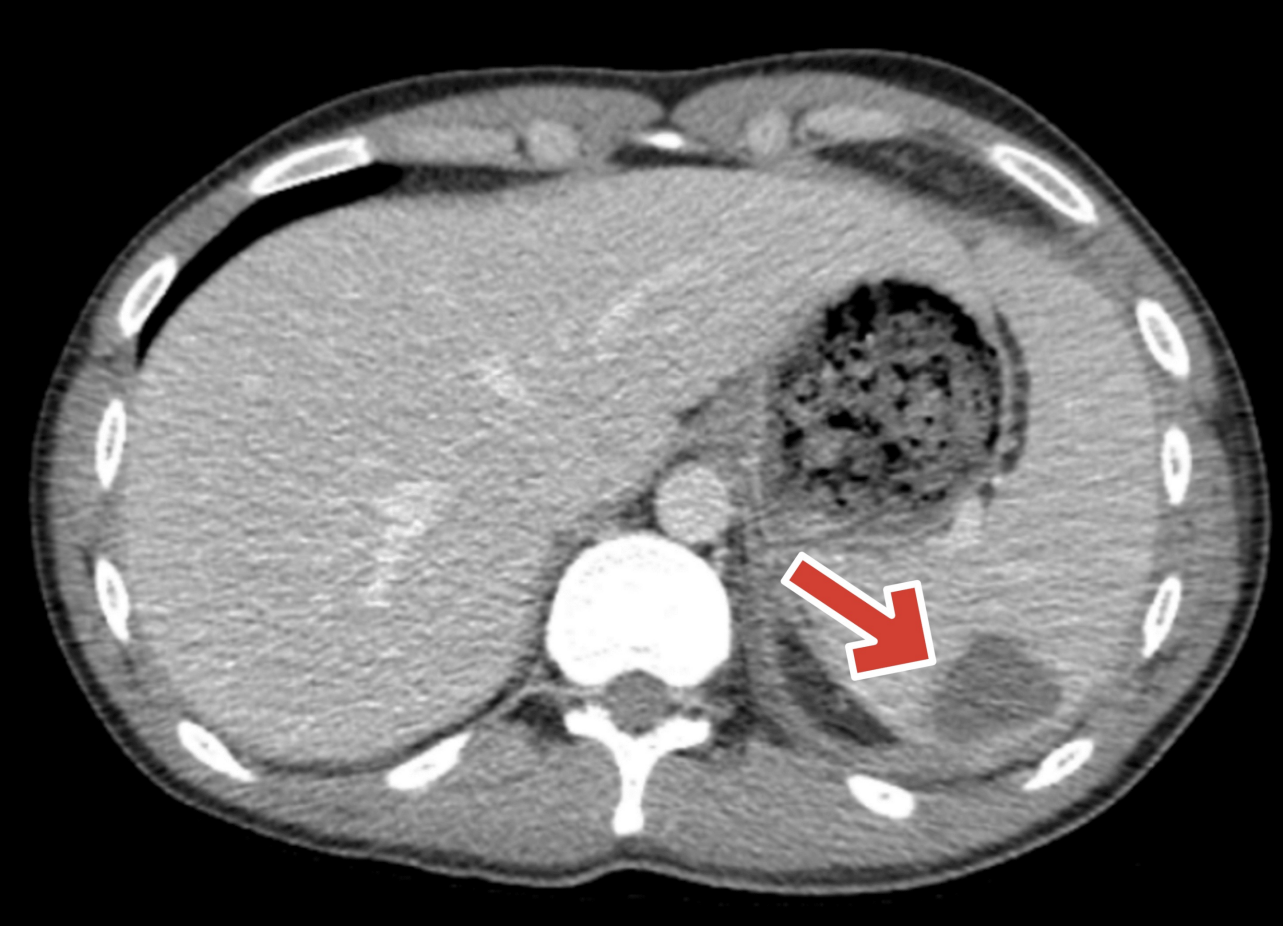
Post-op day 18 CT abdomen/pelvis showing interval decrease in size of splenic collection after drainage (2.2x1.8cm). Arrow pointing at the splenic collection.
